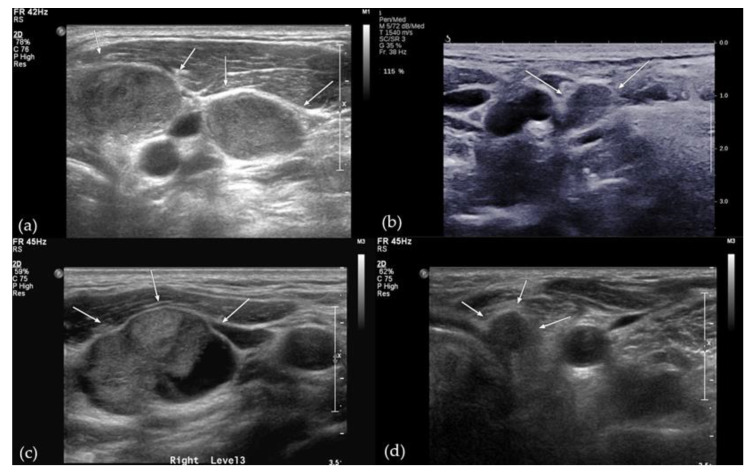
Ultrasound images showing diagnostic features of N1b papillary thyroid carcinoma. (a) Preoperative ultrasound image of a 24-year-old man showing hyperechoic metastatic lymph nodes on the left side of the neck at level III (arrows). (b) Follow-up ultrasound image taken 51 months after surgery, showing an 11-mm irregular, hyperechoic lymph node on the right side of the neck (arrows), which was a recurrence. (c) Preoperative ultrasound image of a 62-year-old man showing a 29-mm metastatic lymph node with hyperechogenicity and cystic change on the right side of the neck at level III (arrows). (d) Follow-up ultrasound image taken 48 months after surgery, showing an 8-mm irregular lymph node on the left side of the neck at level VI (arrows), which was confirmed to be a recurrence.
